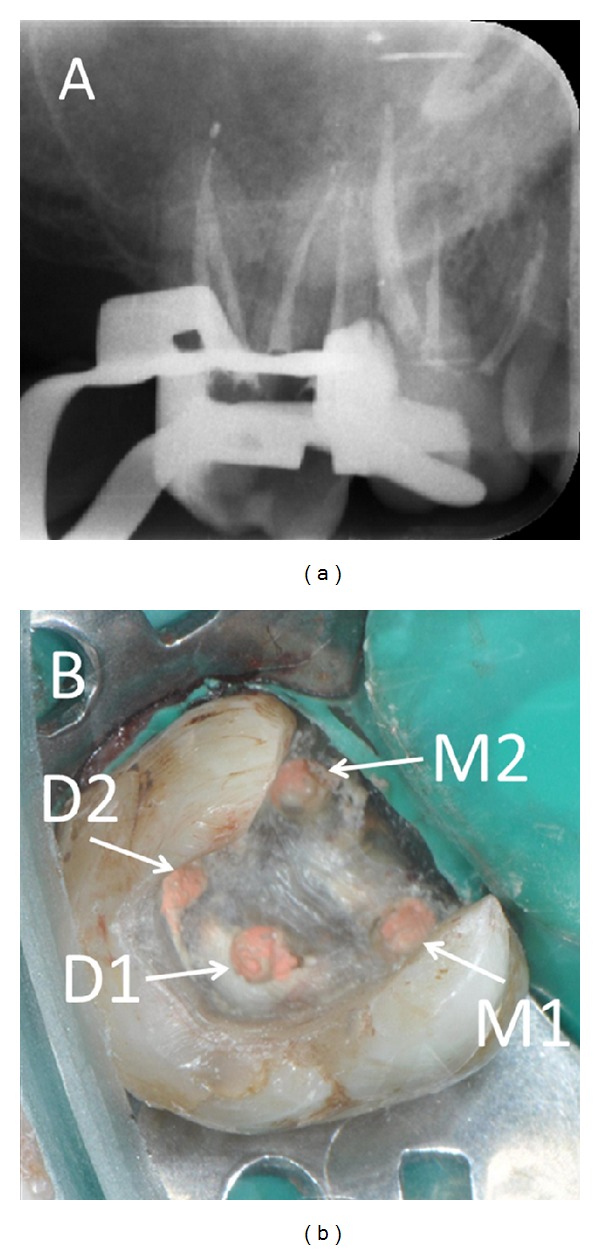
(a) Postoperative periapical radiograph taken immediately after completion of the root canal treatment. (b) The location of four root canal orifices after root canal filling.
